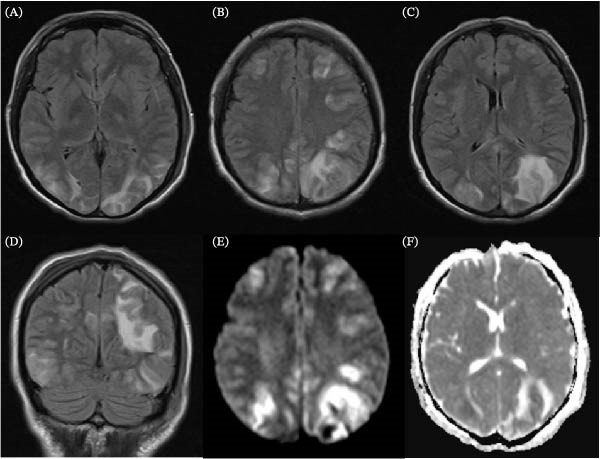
MRI brain with contrast. (A, B) Axial T2 FLAIR shows multiple areas of cortical and subcortical vasogenic edema most prominent in the parietal and occipital lobes and, to a lesser extent, the frontal lobe. (C, D) Axial and coronal T2 FLAIR identified a hyperintense area of acute hemorrhage in the left parietal lobe with surrounding vasogenic edema and mass effect. (E, F) Axial DWI also demonstrates vasogenic edema as a hyperintense signal.
